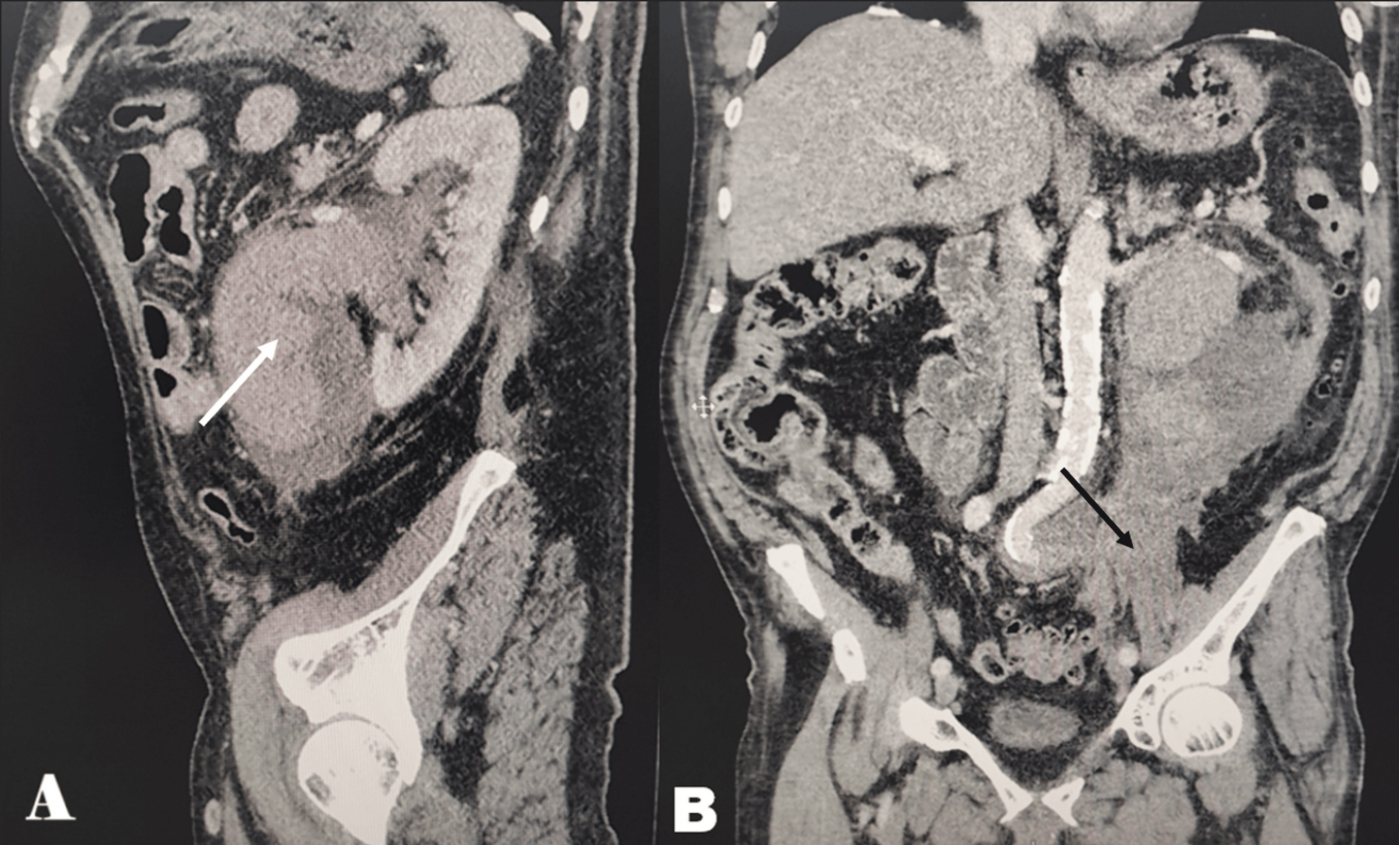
CT scan of the pelvis A: The white arrow shows hyperdense content mixed with fluid density. B: The lesion can be traced to the pelvis as pointed by the black arrow.
